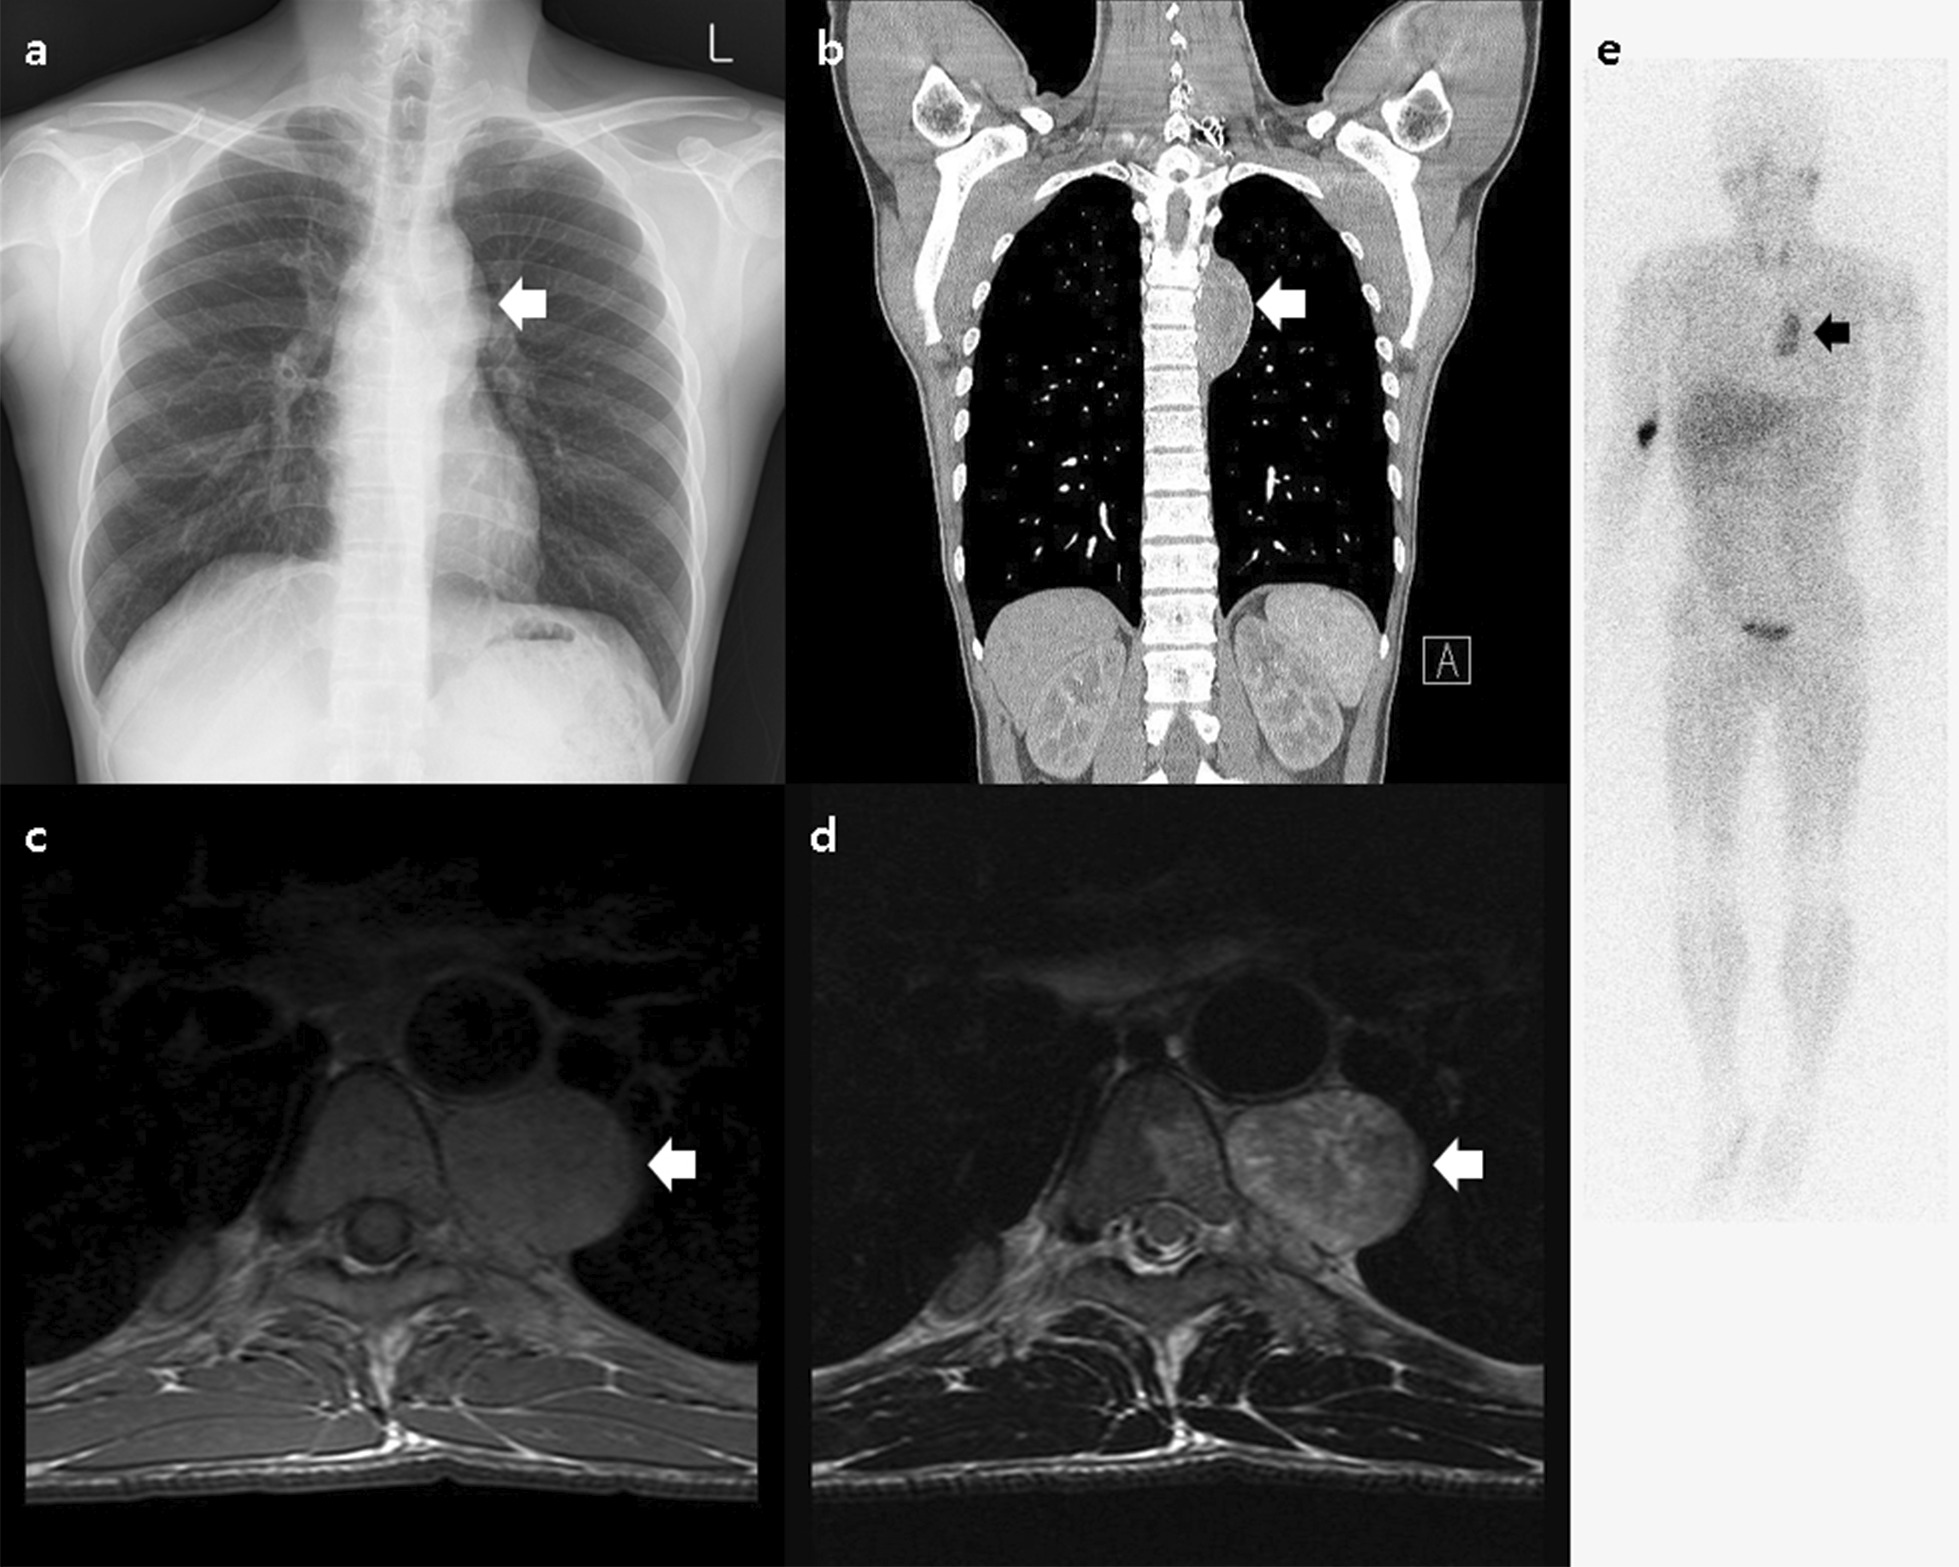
a Chest radiograph showing a mass-like lesion (white arrow) near the left hilum. b Coronal section of a contrast-enhanced chest computed tomography showing a 7-cm heterogeneous mass (white arrow) on the left side of the vertebra (T4-6 level). c and d Magnetic resonance imaging revealed that the paravertebral mass (white arrow) had intermediate signal intensity on the T1-weighted axial image (c) and high signal intensity on the T2-weighted image (d). e An ^123^I-metaiodobenzylguanidine scanning showing focally high tracer uptake in the left paravertebral area (black arrow)
